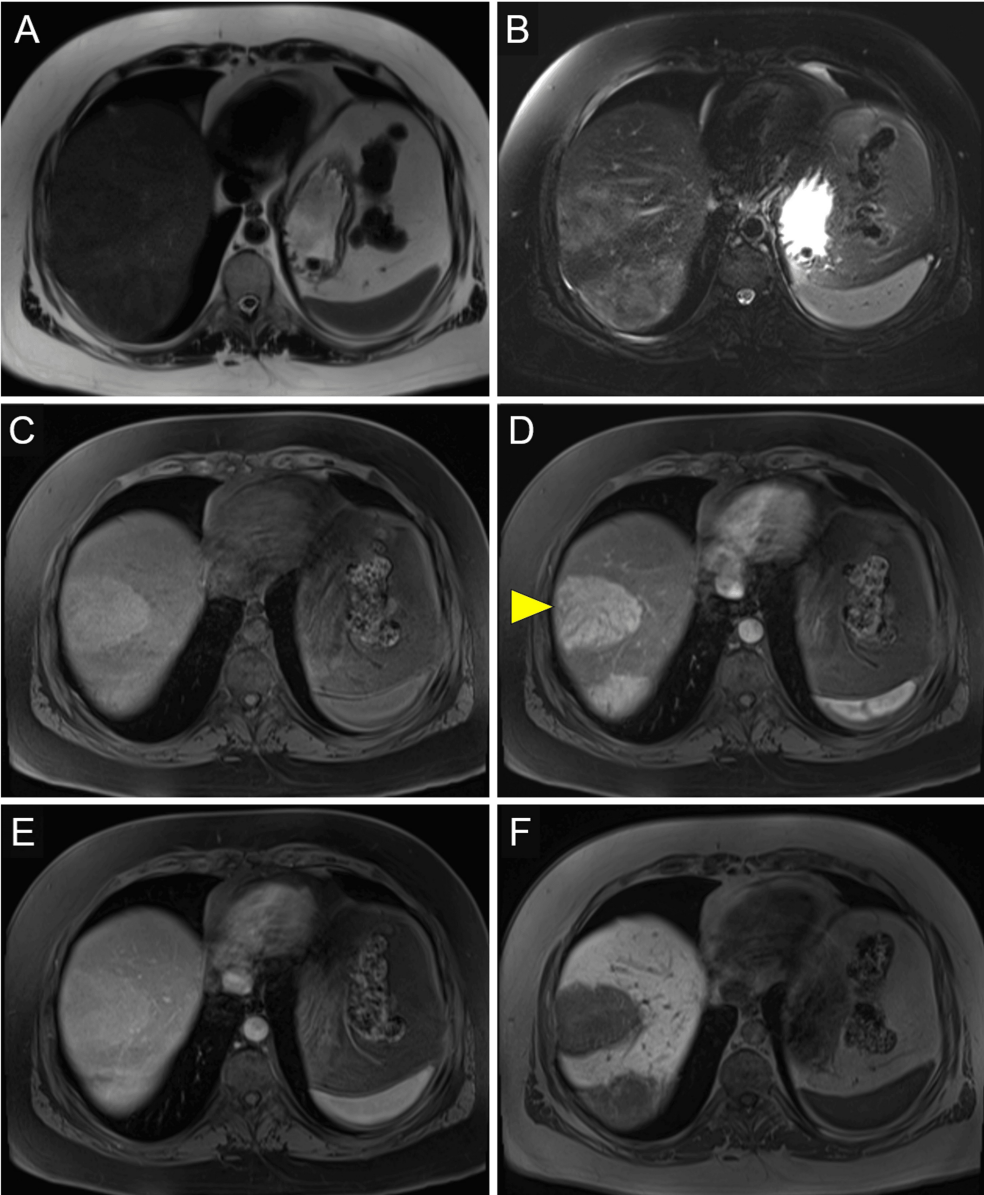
Hepatic adenoma segment VIII Hepatic adenoma in segment VIII measuring 6.7 x 5.5 cm (arrowhead). MRI sequences include T2-weighted (A), T2-weighted with fat saturation (B), and T1-weighted images with fat saturation obtained over multiple phases, including pre-contrast (C), post-contrast arterial phase (D), venous phase (E), and hepatobiliary phase (F).
